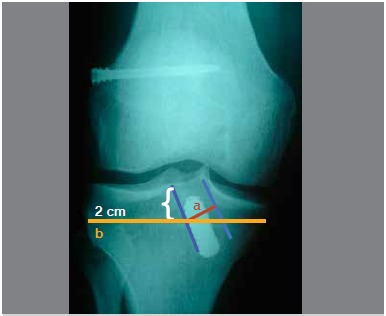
Anteroposterior radiography (AP). The blue line follows the edges of the tunnel and at 2 cm below the medial joint line, perpendicular thereto, its diameter is represented by the line "a". At the same point the diameter of the bone is calculated, represented by line "b". The value obtained by the variable "a" is then divided by the constant.
